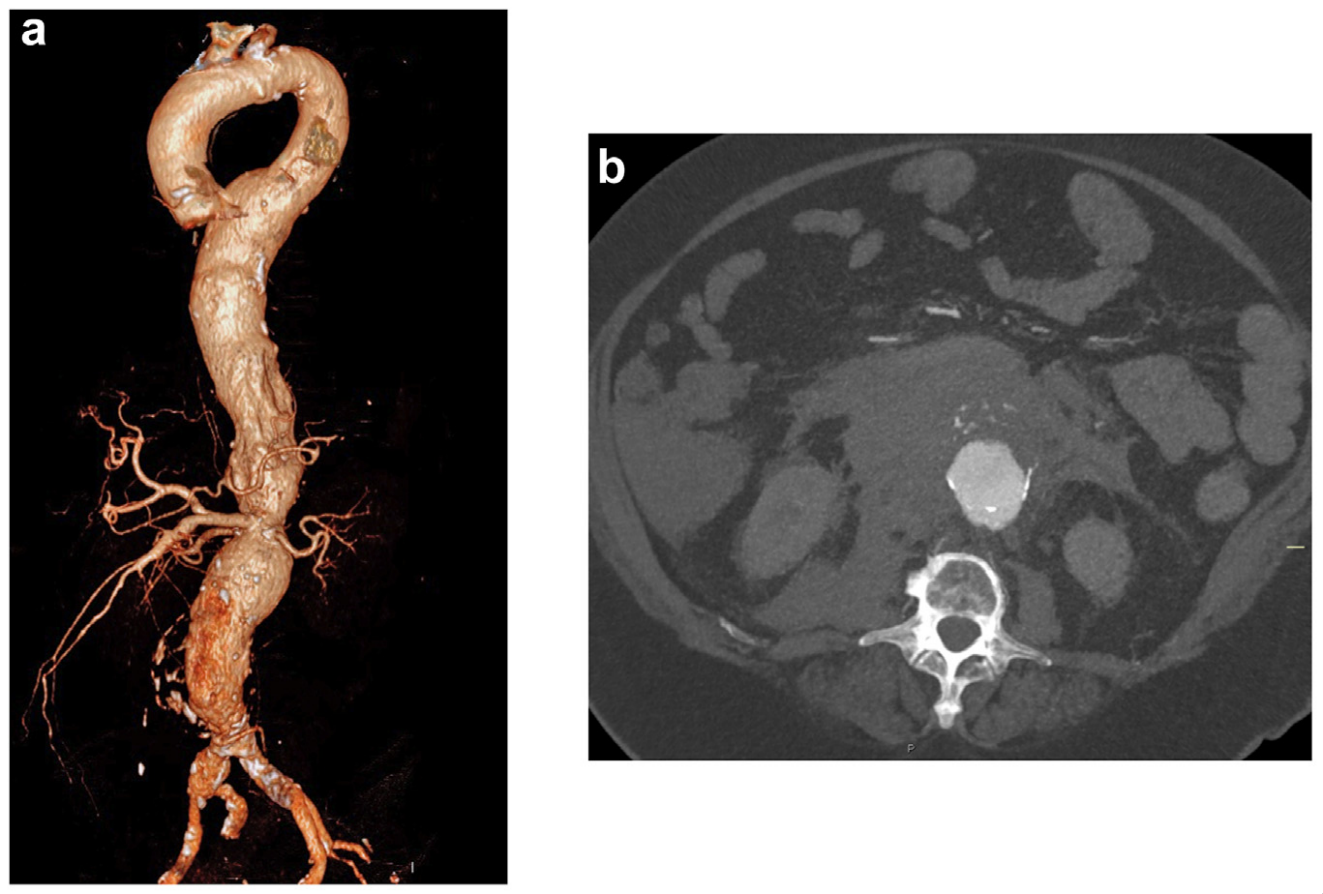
a, Three-dimensional reconstruction of computed tomography scan demonstrating a Crawford type I thoracoabdominal aortic aneurysm (TAAA) and a concomitant tandem abdominal aortic aneurysm (AAA). b, Axial view of computed tomography angiogram demonstrating the ruptured aortic aneurysm.
